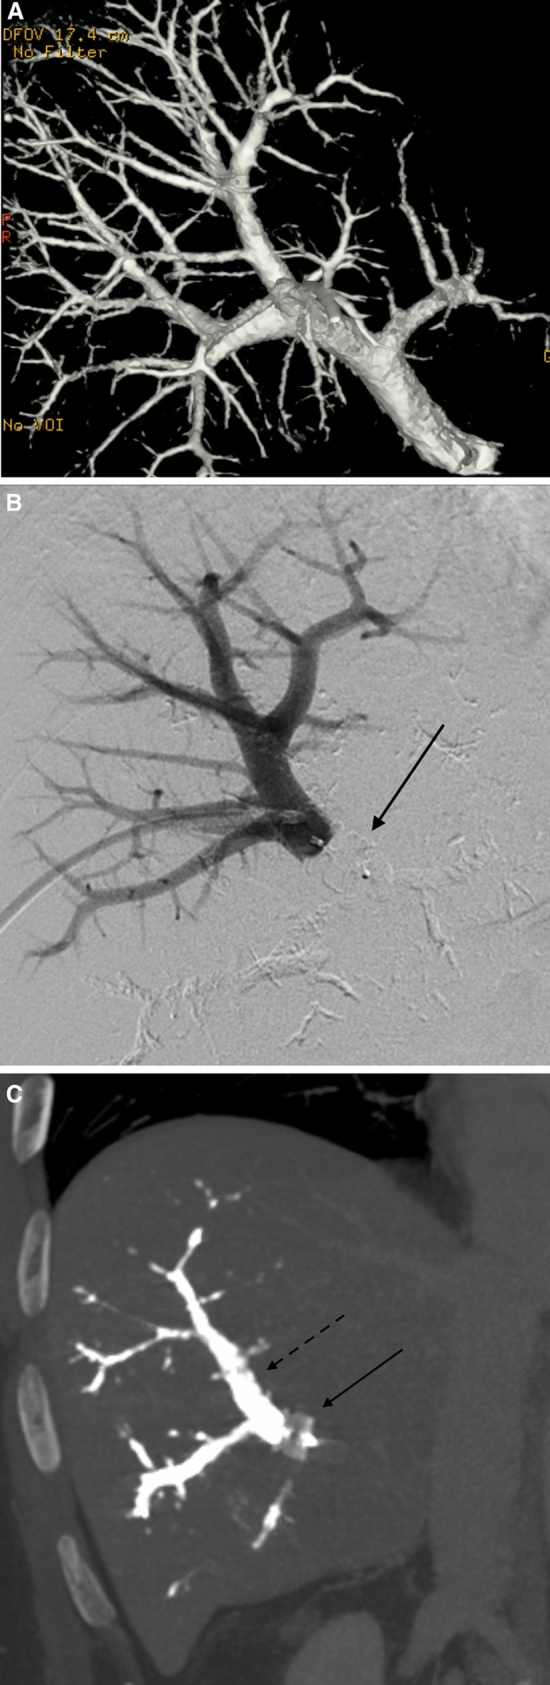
Portal vein embolization in a patient with colorectal liver metastases using a combination of glue and a central vascular plug guided by contrast-enhanced cone beam CT. A. Image of cone-beam CT 3D volume rendering which can be used as real-time overlay during fluoroscopy B. Digital subtraction angiography image after placement of a vascular plug (arrow) in the main right portal vein and into the main anterior sector stem after selective embolization of the posterior sector C. Maximum intensity projection image of contrast-enhanced CT 4 weeks after PVE showing the plug (black arrow) and glue cast (stapled black arrow) in the anterior sector
